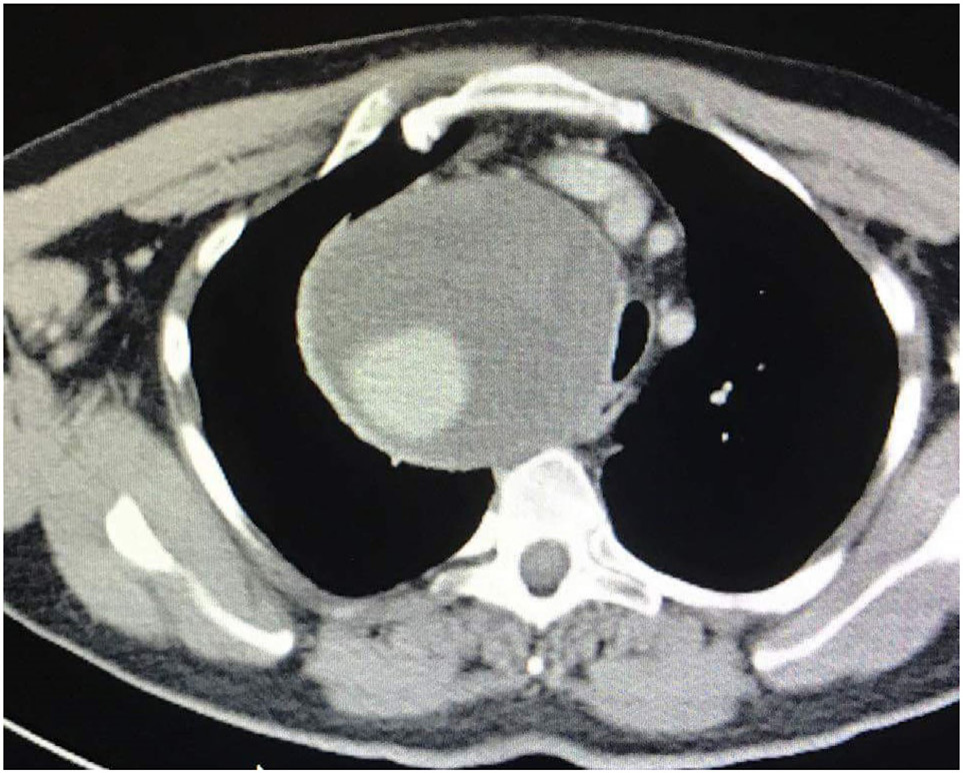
Axial-coronal computed tomography imaging shows a giant right subclavian aneurysm of 12 cm diameter.
